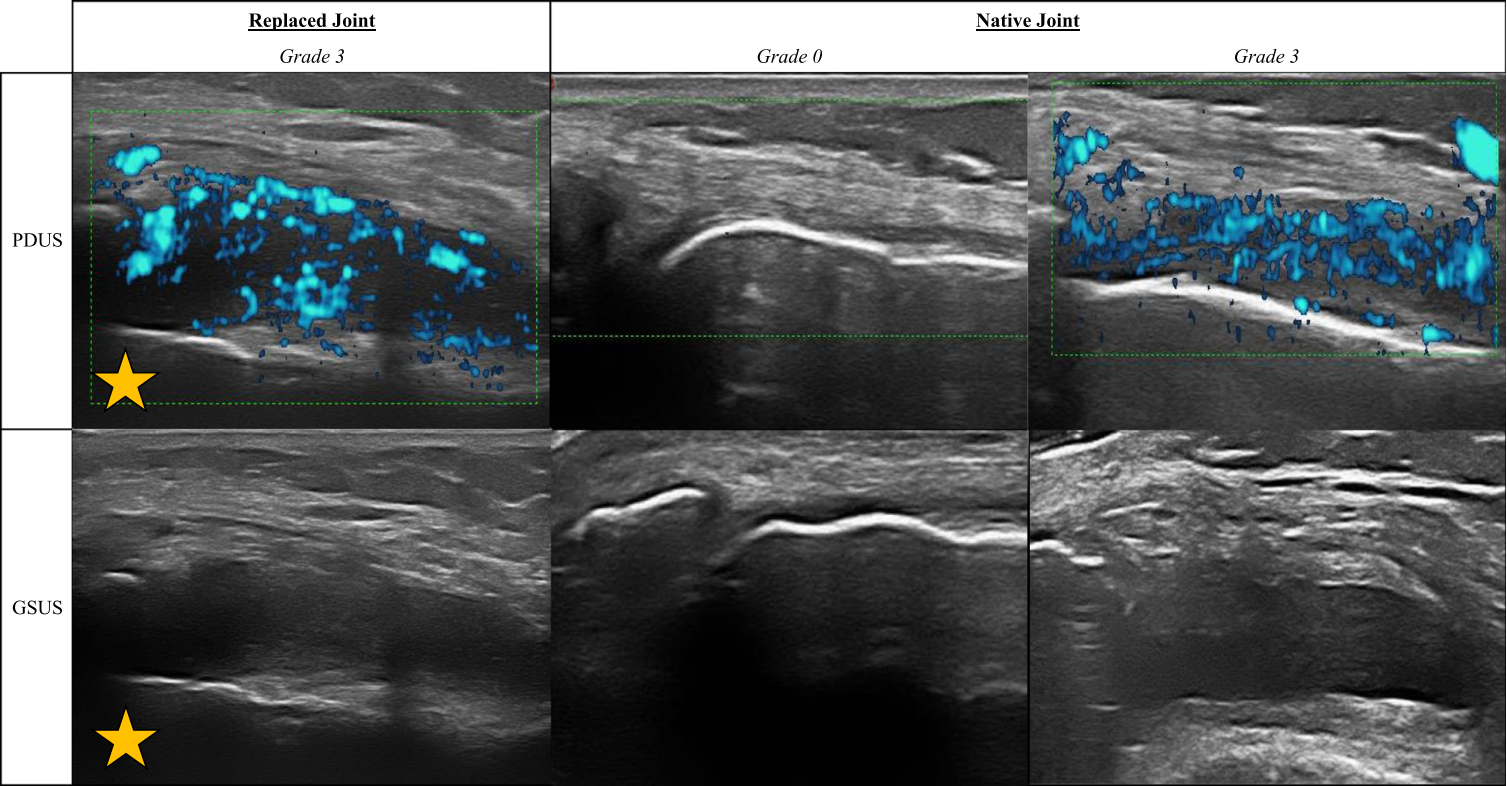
Synovitis detectable by ultrasound of the lateral knee. Left column, Power Doppler ultrasound (PDUS) and grayscale ultrasound (GSUS) of replaced joint, Grade 3 (severe synovitis). Middle column, PDUS and GSUS of native joint, Grade 0 (no synovitis). Right column, PDUS and GSUS of native joint, Grade 3 (severe synovitis). Yellow star indicates the location of the prosthesis
